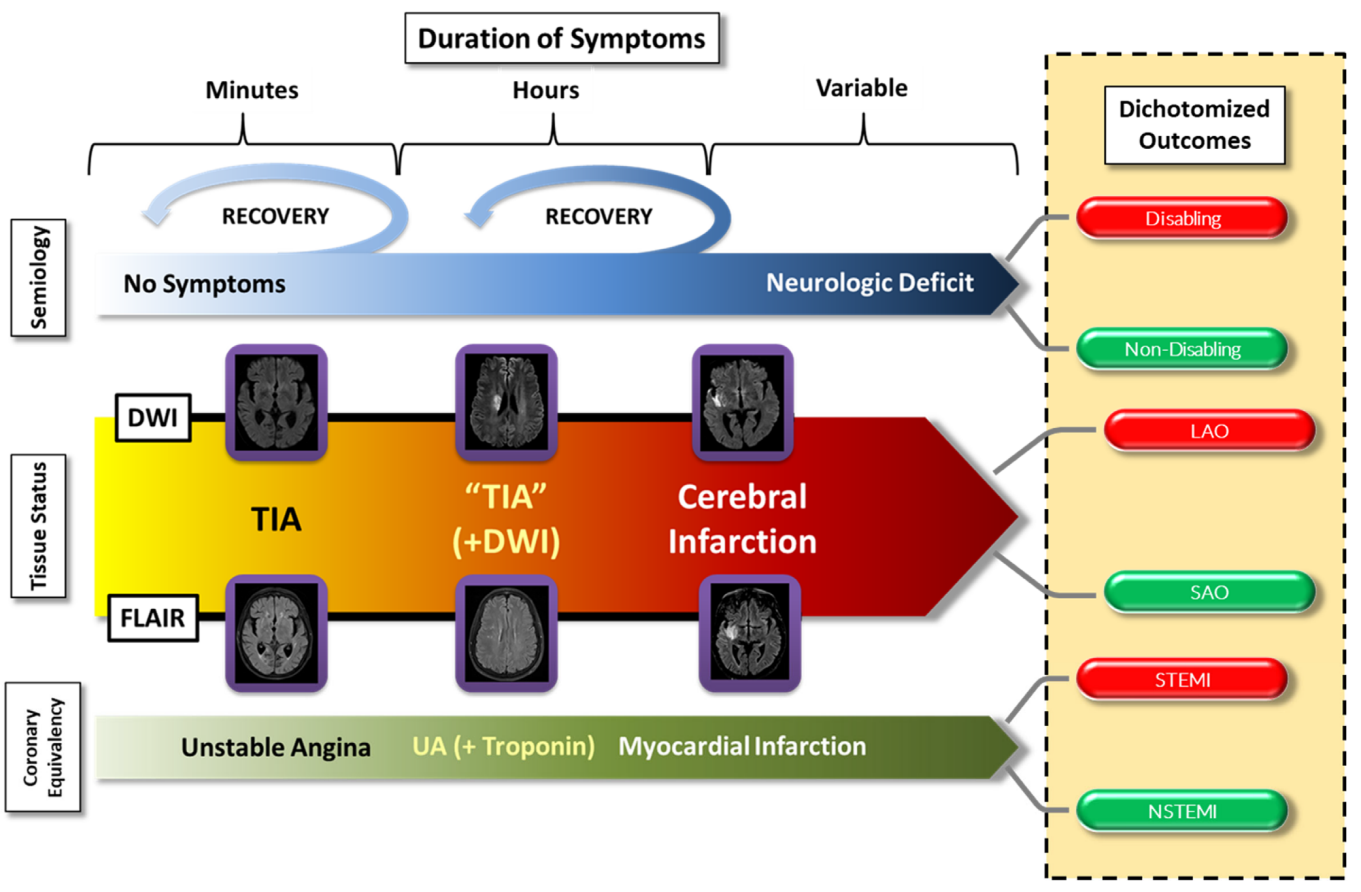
The continuum of acute cerebrovascular syndromes. The incorporation of both clinical aspects (i.e., specific syndrome and duration of symptoms) and the imaging status (i.e., imaging evidence of tissue injury or not) underscores the importance of considering transient ischemic attack (TIA) as part of a continuum rather than a discrete and separate entity from cerebral infarction. It also allows an overall understanding that is comparable to the one widely used for the coronary circulation. In this context, ischemic strokes due to large arterial occlusion (LAO) would be comparable to ST-segment elevation myocardial infarctions (STEMIs), while smaller infarctions would be more analogous to non-STEMIs (NSTEMIs). On another dimension, the presence or absence of a neurologic deficit may or may not be associated with evidence of tissue injury and, depending on the location and volume of the compromised brain tissue, may or may not result in a disabling outcome. See text for further description. DWI, diffusion-weighted imaging; FLAIR, fluid-attenuated inversion recovery; SAO, small arterial occlusion; UA, unstable angina.
